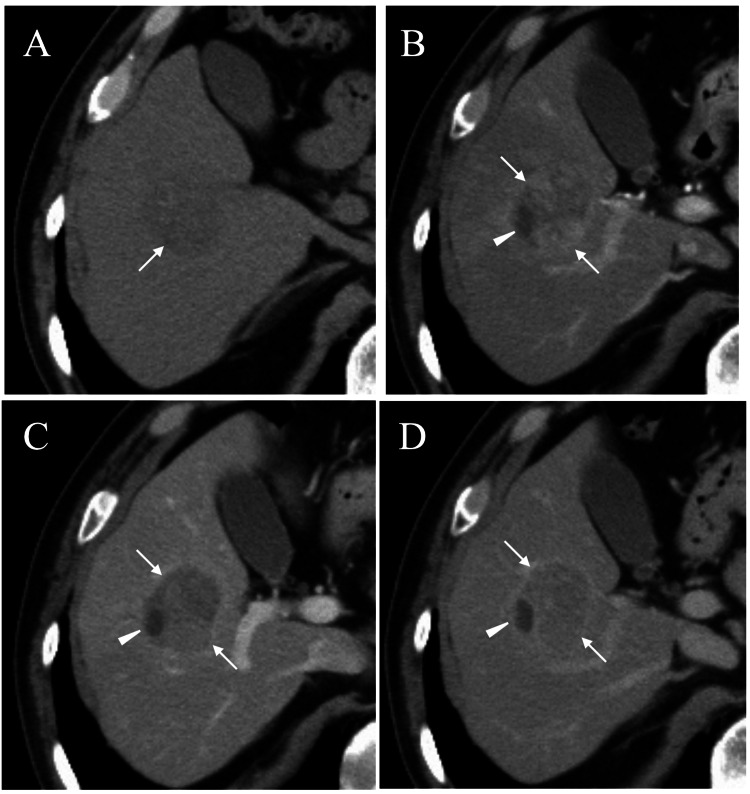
Computed tomography (CT) findings A. CT showed an oval mass (arrow) with a slightly lower CT Hounsfield Unit value compared to that of the surrounding liver parenchyma. B. CT showed a weak enhancement predominantly in the mass margins (arrows) and a small part with no enhancement (arrowhead) on the early arterial phase. C. CT showed a washout pattern (arrows) and a clearly demarcated non-enhancement small area (arrowhead) on the late arterial phase. D. CT showed a retained slight rim enhancement (arrows) and a non-enhancement area (arrowhead) on the portal phase.
